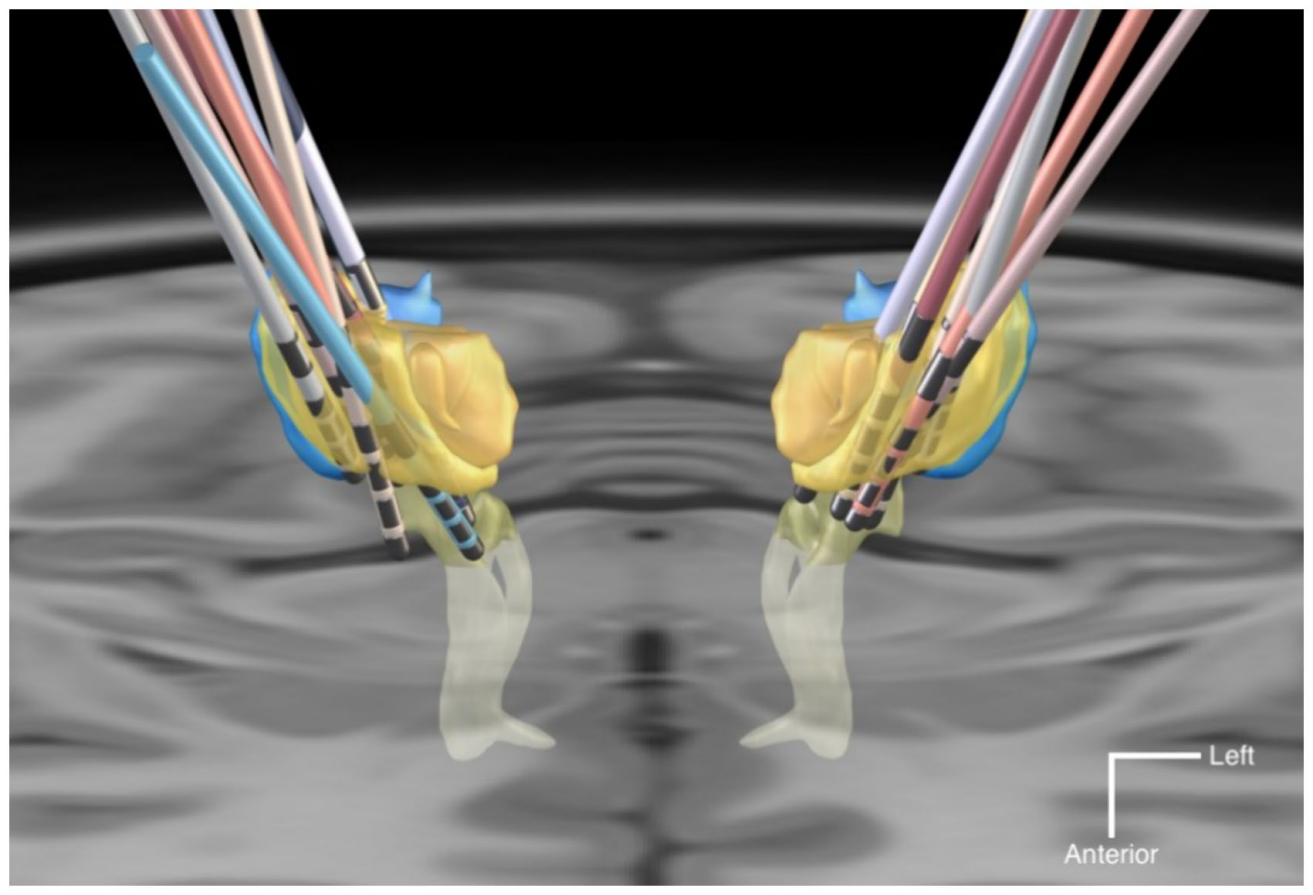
Graphic rendering of the 18 DBS leads with anatomical landmark structures, in a common space. The anatomical structures are sourced from the DISTAL atlas (54). VIM is colored in yellow; VPL/VPM in blue; Medial Lemniscus in light-yellow. The background is an axial T1-MRI slice (z = 0 mm) of the MNI template. The mean coordinates (±SD) of the deepest contact points of the VIM-targeted leads were *x* = −12.6 (±2.1), *y* = −19.8 (±1.8), *z* = −3.9 (±1.8) in the left hemispheres, and *x* = 13.6 (±1.6), *y* = −19.6 (±1.7), *z* = −3.5 (±1.9) in the right hemispheres. For the VPL-targeted leads these were *x* = 10.1 (±0.4), *y* = −24.2 (±1.7), *z* = −7.8 (±0.9).
